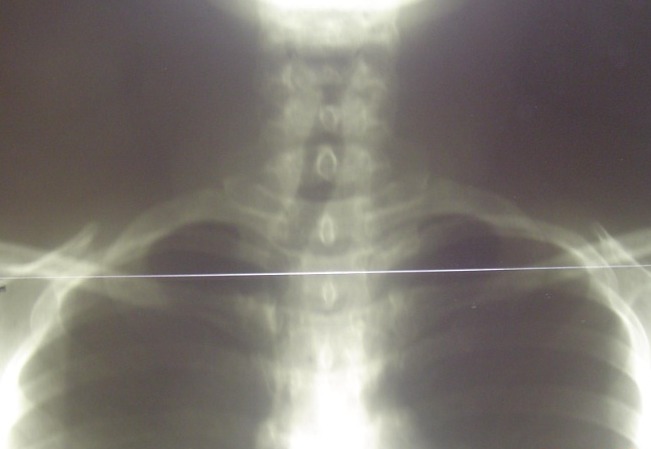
Radiographie pulmonaire de face montrant le refoulement de la trachée en dehors et à droite par cette masse latérocervicale gauche
